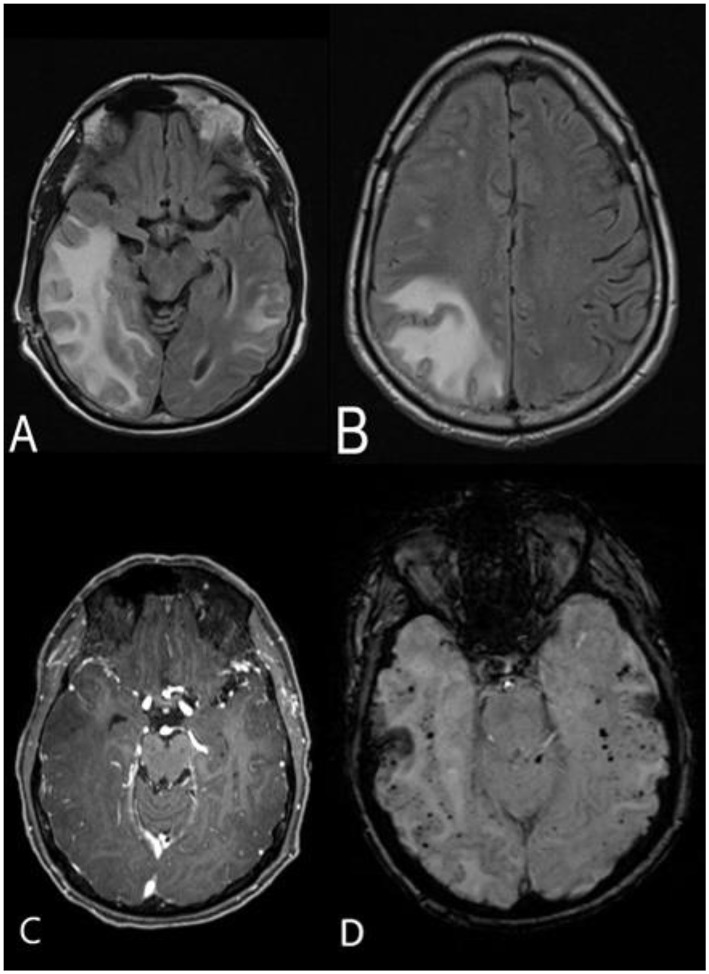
MRI brain without and with contrast approximately 3 weeks after the initial scan. Axial FLAIR sequence demonstrates significant progression of the hyperintense lesions in the right temporal, occipital, and fronto-parietal areas, with mild mass effect on the right lateral ventricle and midline shift (A,B). No contrast enhancement is appreciated (C). SWI sequence shows stable, diffuse, bihemispheric microhemorrhages (D).
